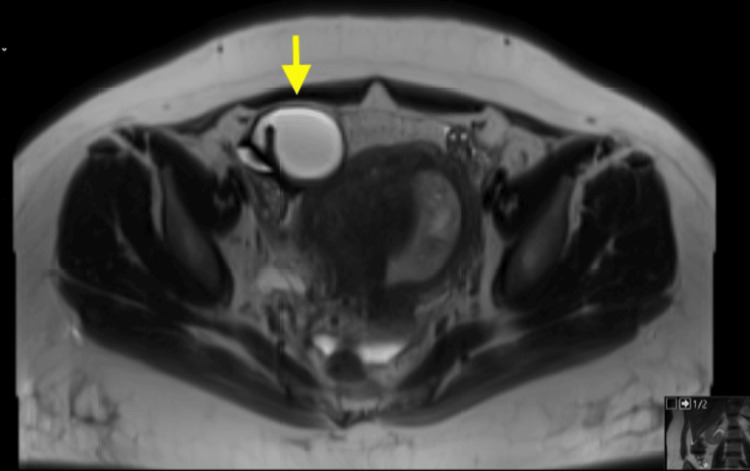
Axial T2 weighted pelvic MRI without contrast identifying a right adnexal cyst (yellow arrow) measuring 4 cm.
